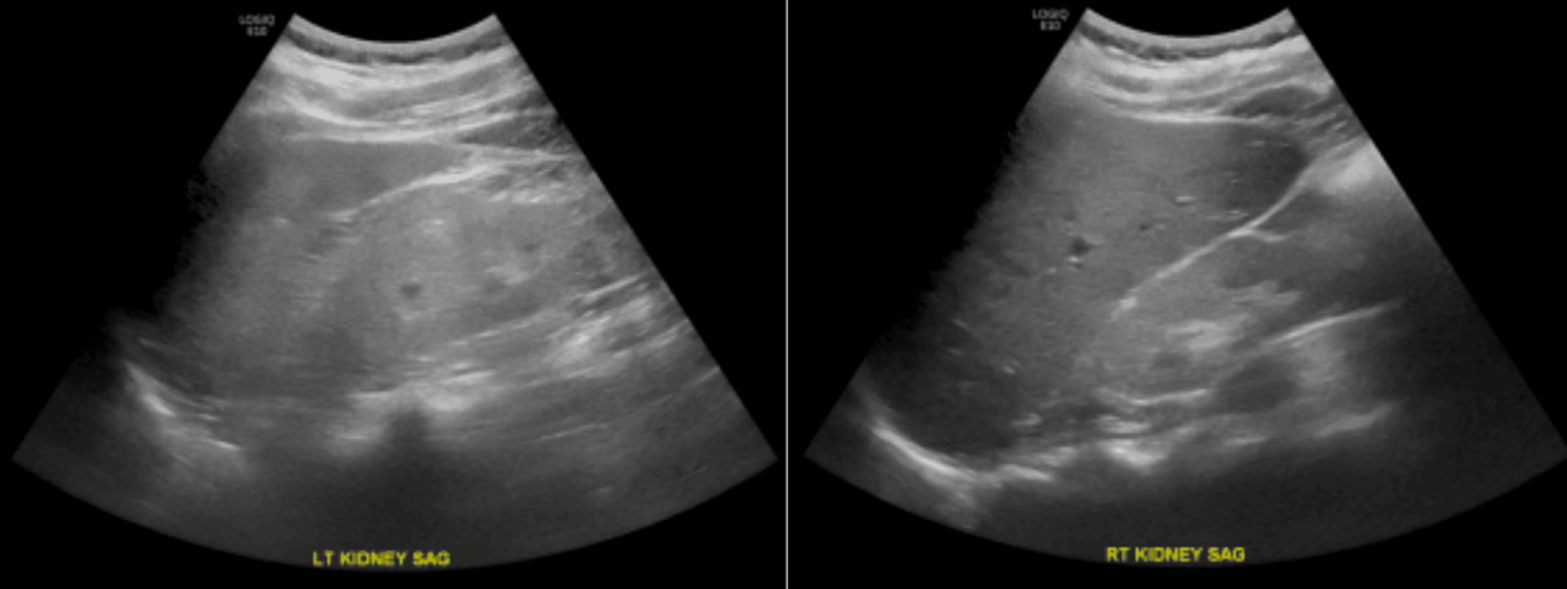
Renal ultrasound of the right and left kidneys showing kidney size with no appreciable hydronephrosis.
